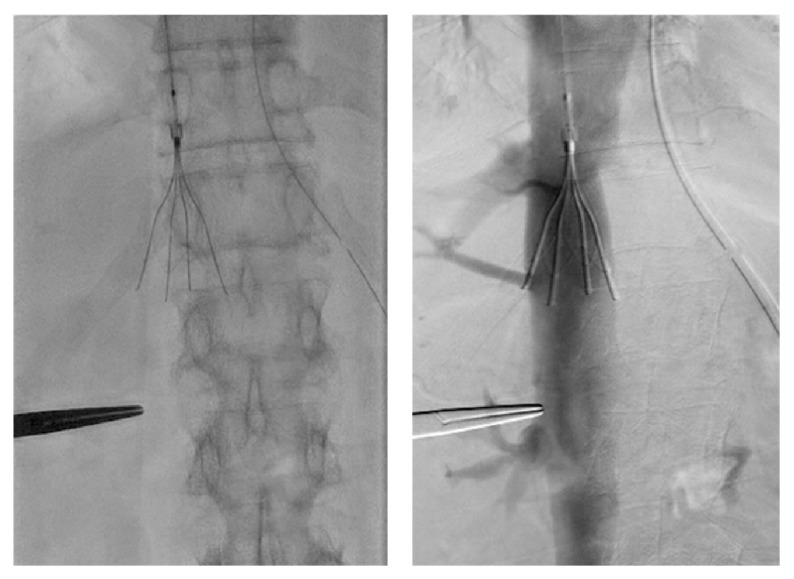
A transient IVC filter was implanted at the site of the suprarenal IVC via the right jugular vein. Insertion of the IVC filter was performed under angiographic and echocardiographic guidance.
